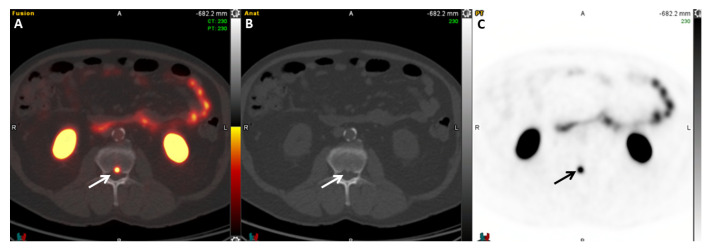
A 69-year-old man with biochemically recurrent prostate cancer with a PSA of 0.1 ng/mL. Fused axial PET/CT (A), axial CT (B), and PET (C), ^18^F-DCFPyL PSMA images demonstrate a focus of increased radiotracer uptake in L2 (SUVmax = 19.1), compatible with a bone metastasis (arrows).
